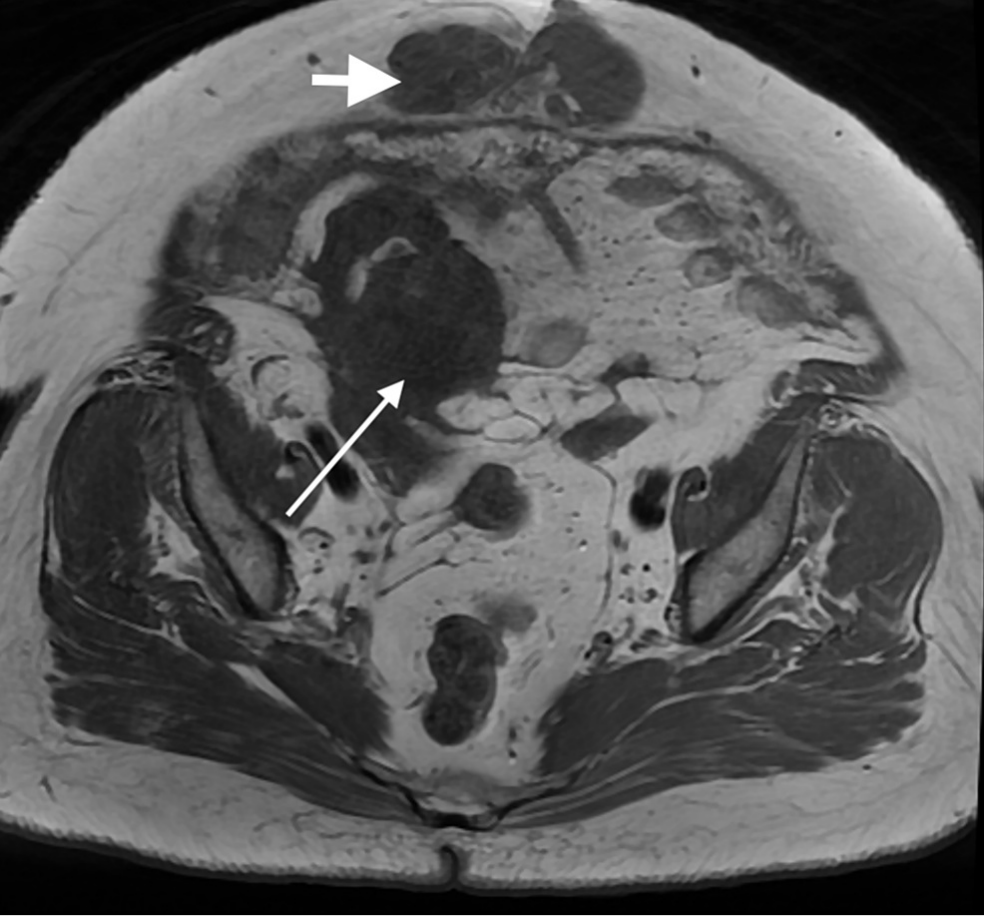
MRI of the abdomen and pelvis in an axial T1WI sequence. An irregularly-shaped low signal intensity structure is seen, corresponding to the lesions seen on the CT images (long arrow). Another low signal lesion is seen at the subcutaneous tissue of the mid abdomen representing the herniated component (short arrow).
